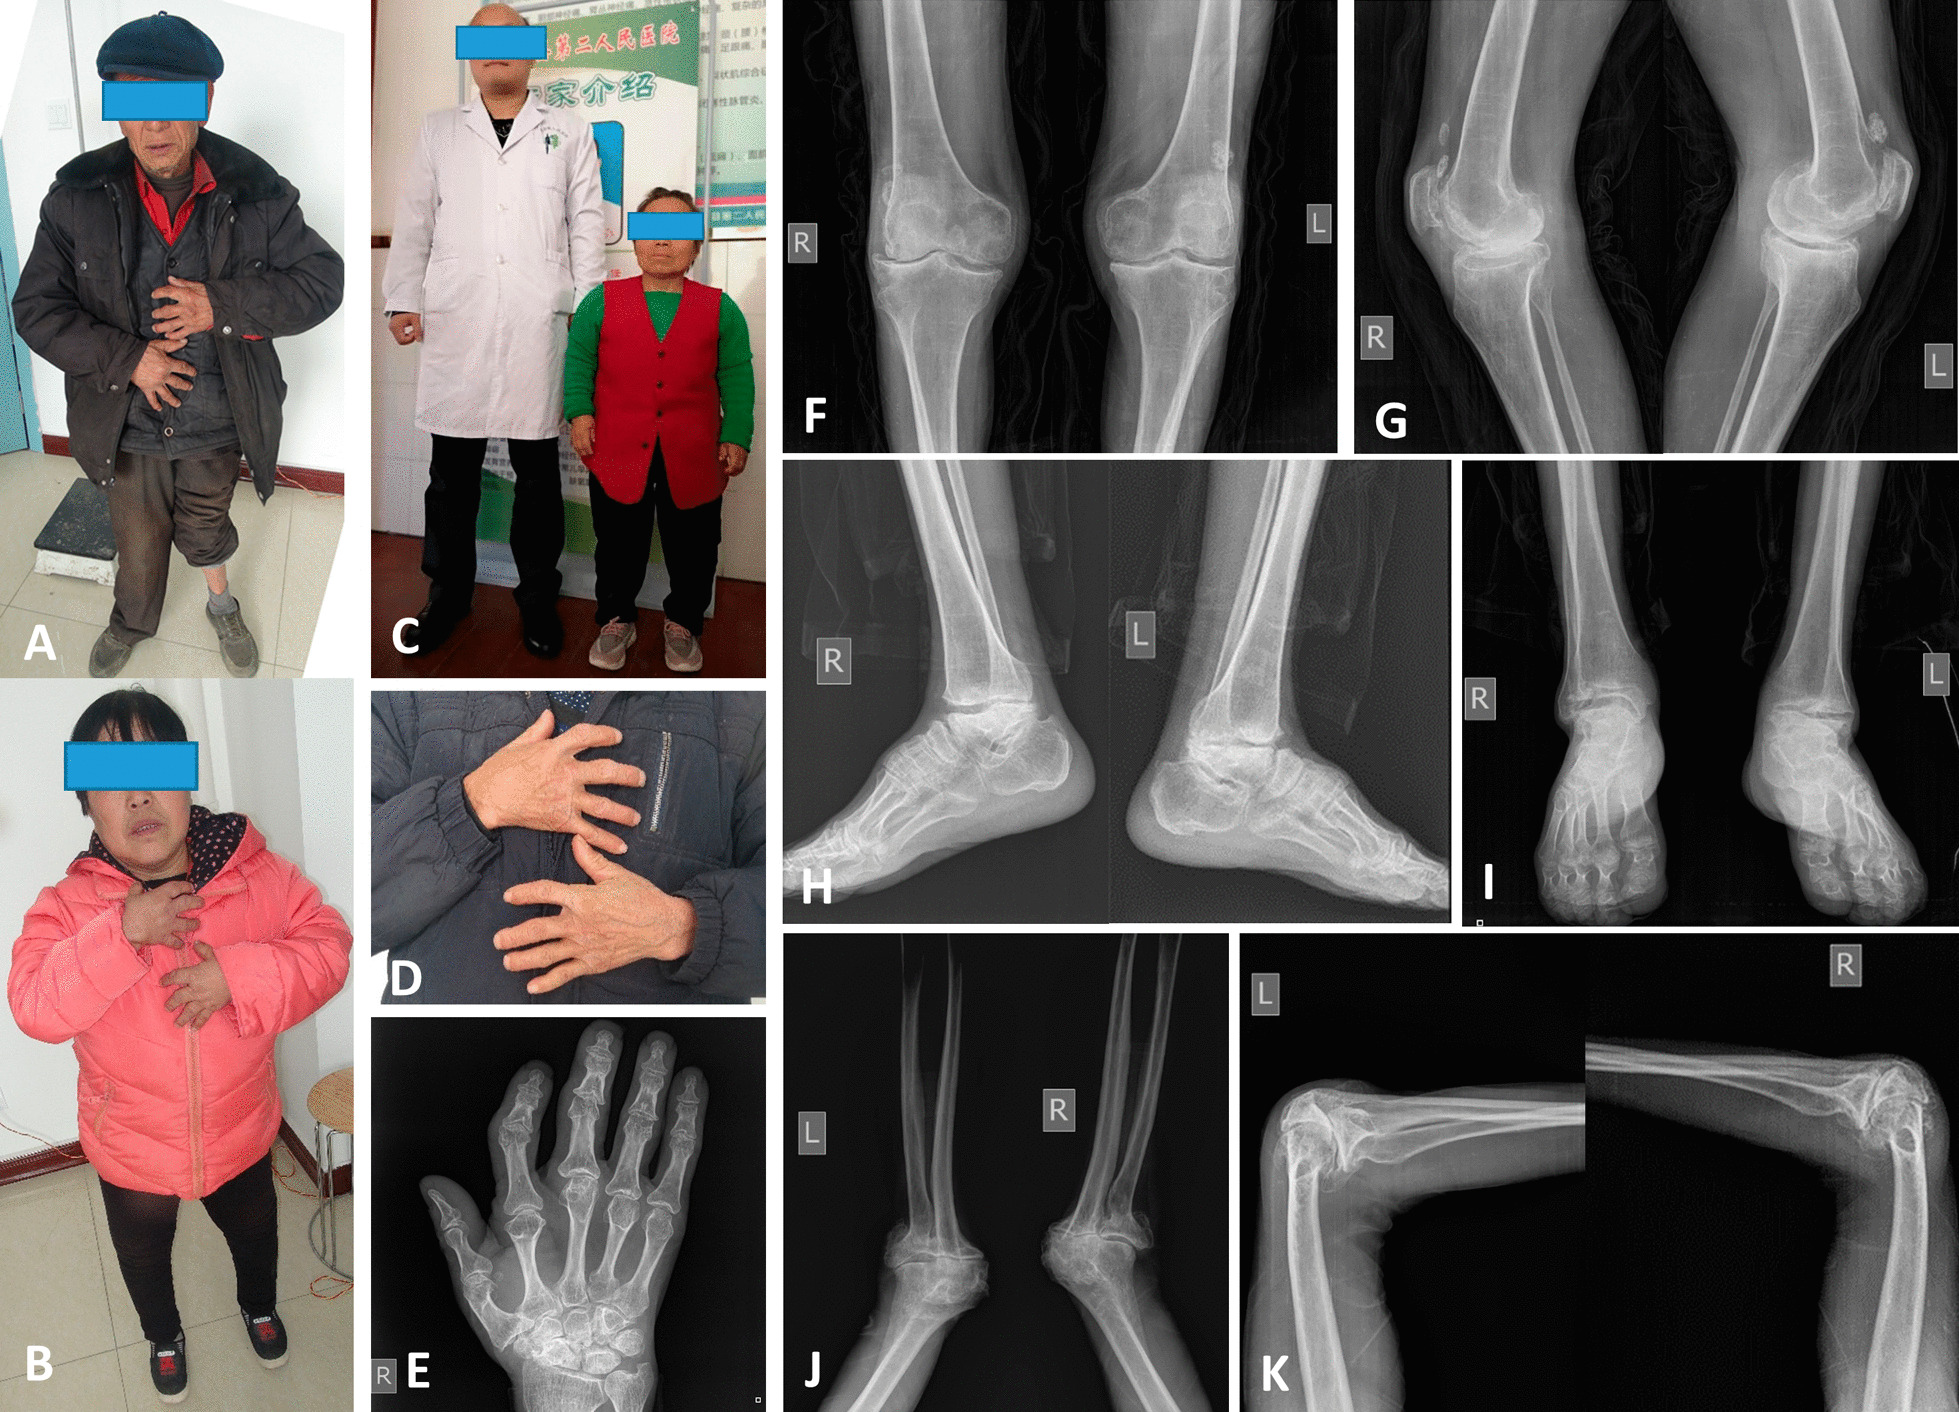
The physical characteristics and joint X-ray features of KBD patients. A The KBD patient presented with severe short deformity of the finger joint, osteophyte formation, and valgus deformity of knee joint. B This KBD patient presented with severe short finger joint deformity and knee varus deformity. C The KBD patient wearing a red coat shows shorter stature than a normal adult with a white coat. D A KBD patient shows a deformity of the finger joint. E X-ray of the hand of KBD patient showed thickening of the finger joints and osteophyte formation. F and G Radiographs of the knee joint in KBD patients showed joint space stenosis, arthrofacial osteosclerosis, and osteophyte formation. H and I Radiographs of the ankle in KBD patients showed narrowing of the ankle space, necrotic collapse of talus, and enlargement of the ankle joint. J and K Radiographs of the elbow in KBD patients showed valgus deformity, joint space narrowing, and osteophyte formation
